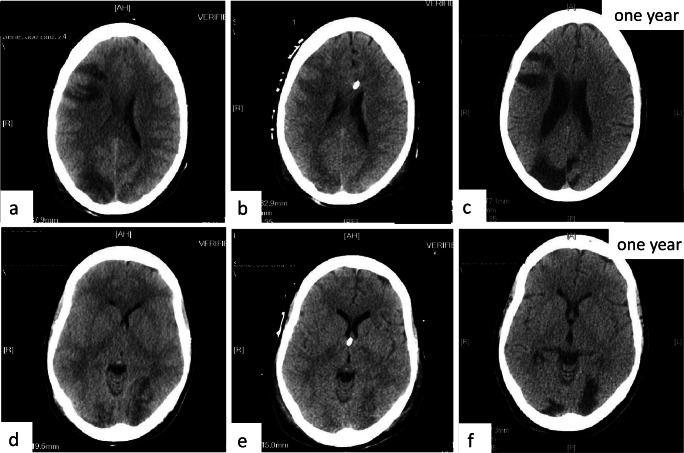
CT images showing two axial plane sections of the brain of patient 4 before and after AF treatment. a, d Day 9 after the trauma when ICP peaked at levels > 30 mm Hg, immediately before the insertion of an EVD (cf. Fig. 1d). Signs of general brain swelling causing a mass effect with obliterated cerebral sulci and compressed ventricles are present in addition to the focal, hypodense, ischemic areas. b, e Day 14, 70 h after the start of Salovum® administration. Sulci are visible and the compressed ventricles have opened up. The focal, hypodense areas have faded. c, f Follow-up 1 year after the trauma
